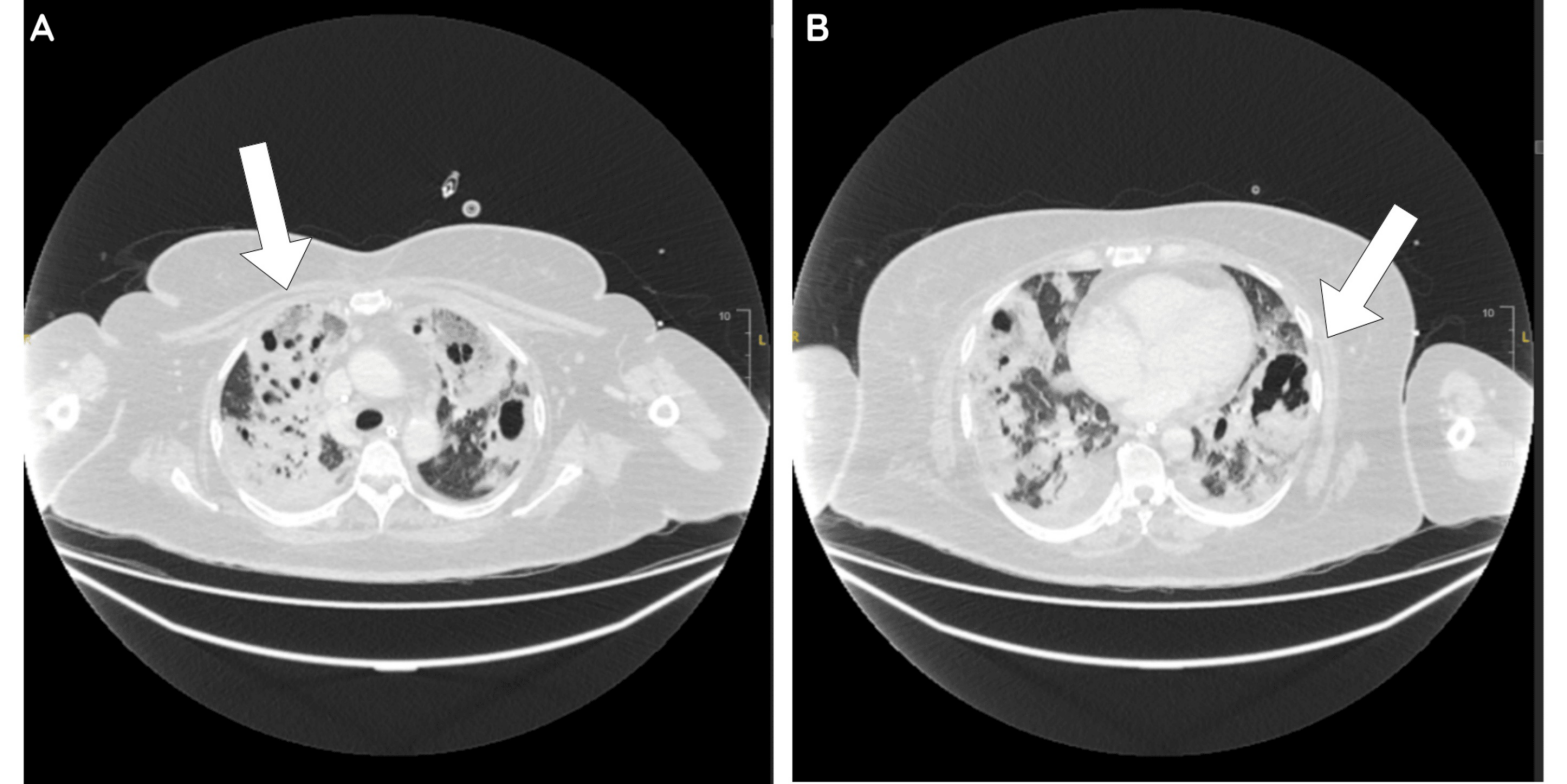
Computed tomography (CT) scan of the chest showing dense bilateral cavitating pulmonary consolidations, with arrows pointing to the cavities. Figure A shows multiple cavities in the right upper lobe, and Figure B depicts cavities in the left lower lobe.
